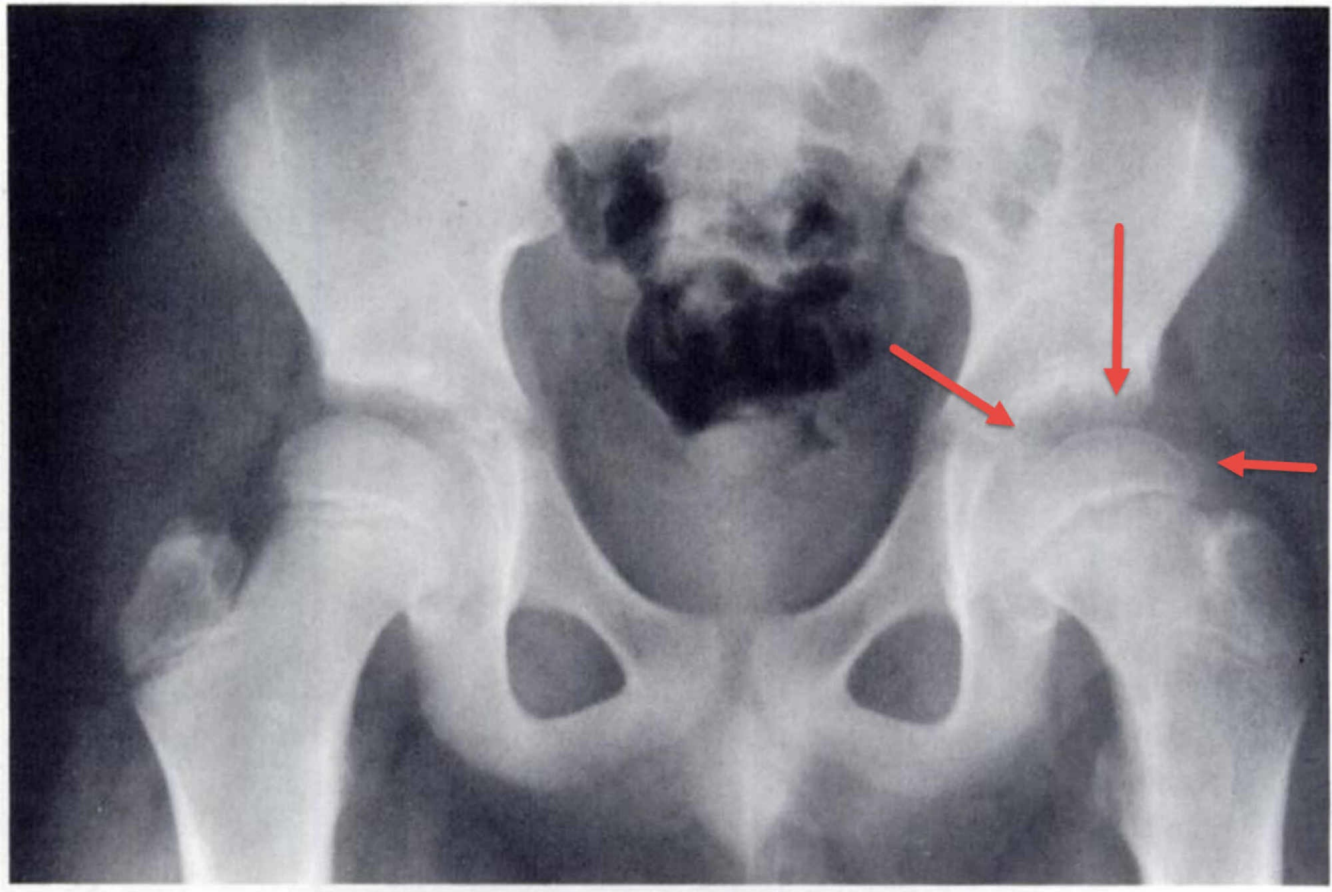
Crescent moon sign in a child with slipped capital epiphysis who was treated with a hip spica A plain radiograph of an eight-year-old child with a slipped capital femoral epiphysis who was treated with a hip spica. The red arrows point toward the subchondral crescent-like osteopenia (moon crescent sign). The patient did not develop avascular necrosis of the femoral head.
